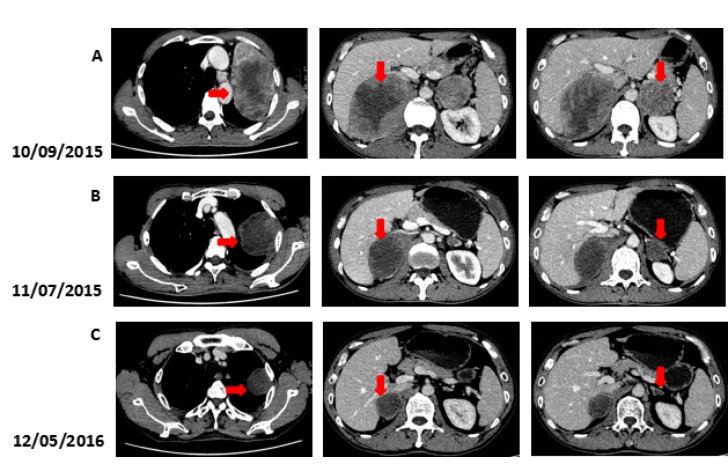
Computed tomography (CT) findings (A) The CT scan shows the lesion in left lung and bilateral adrenal (arrows). (B, C) A restaging CT scan shows the lesion in left lung and bilateral adrenal keep shrinking after two, fifteen courses of Nivolumab (arrows).
